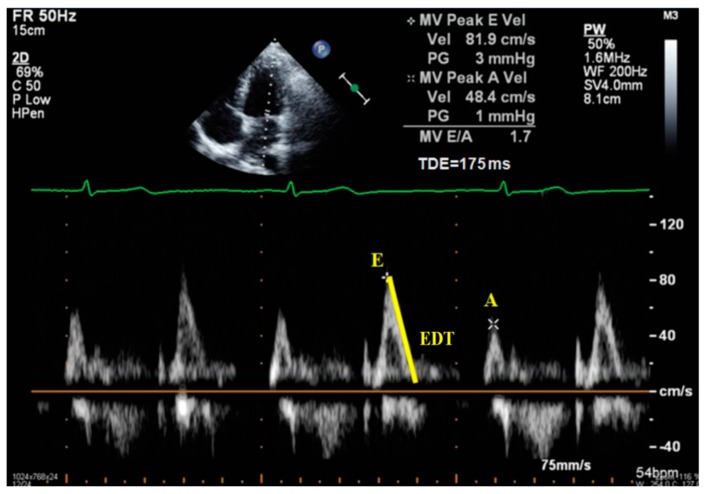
Echocardiographic assessment of mitral flow with pulsed Doppler in a patient in sinus rhythm. A: A wave velocity (cm/s); E: E wave velocity; EDT = E wave deceleration time.
